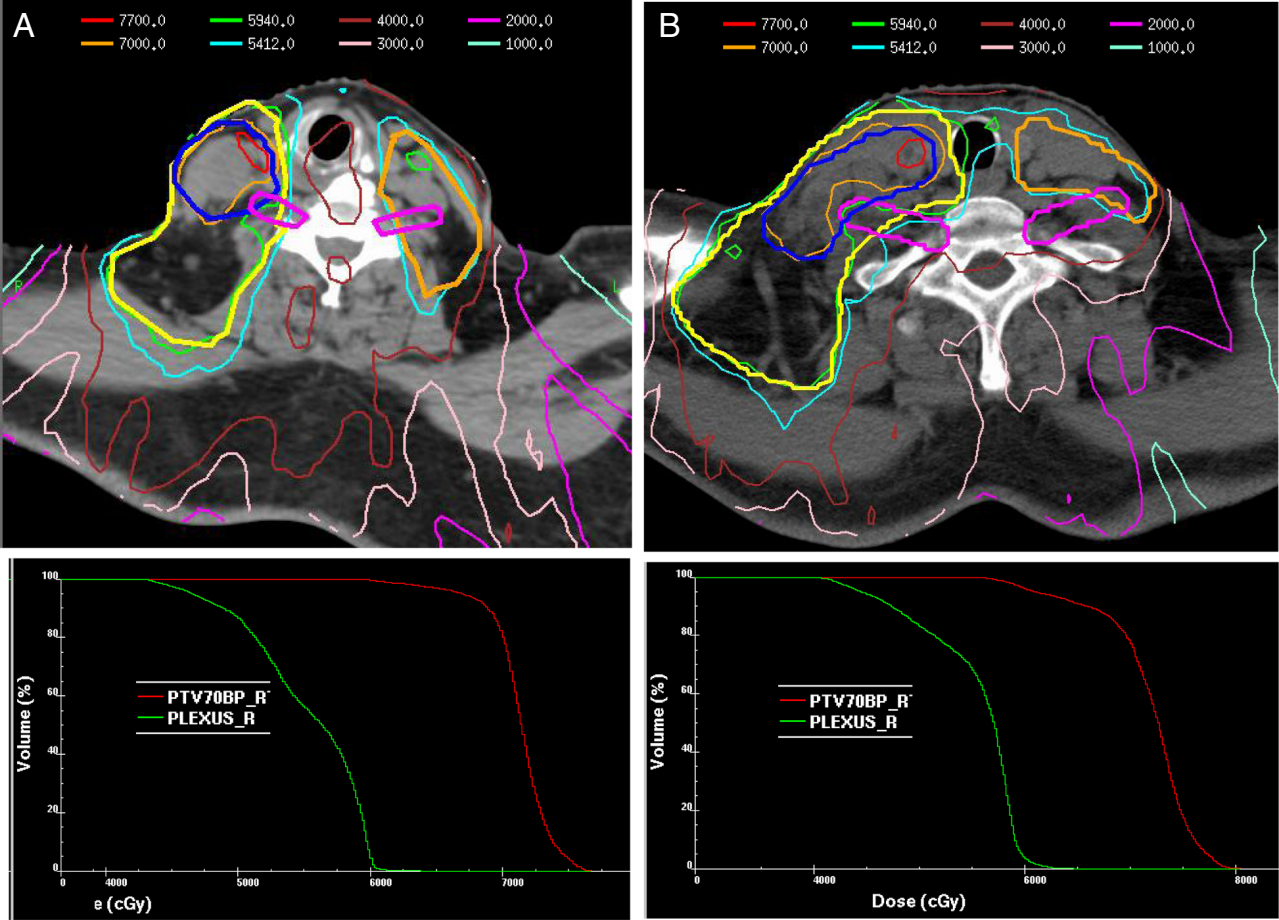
A. and B. CT slices through the low neck at the level of the brachial plexus along with corresponding dose-volume histograms in two representative patients demonstrating constraining of the brachial plexus at the expense of the immediately adjacent gross nodal disease PTV coverage.
